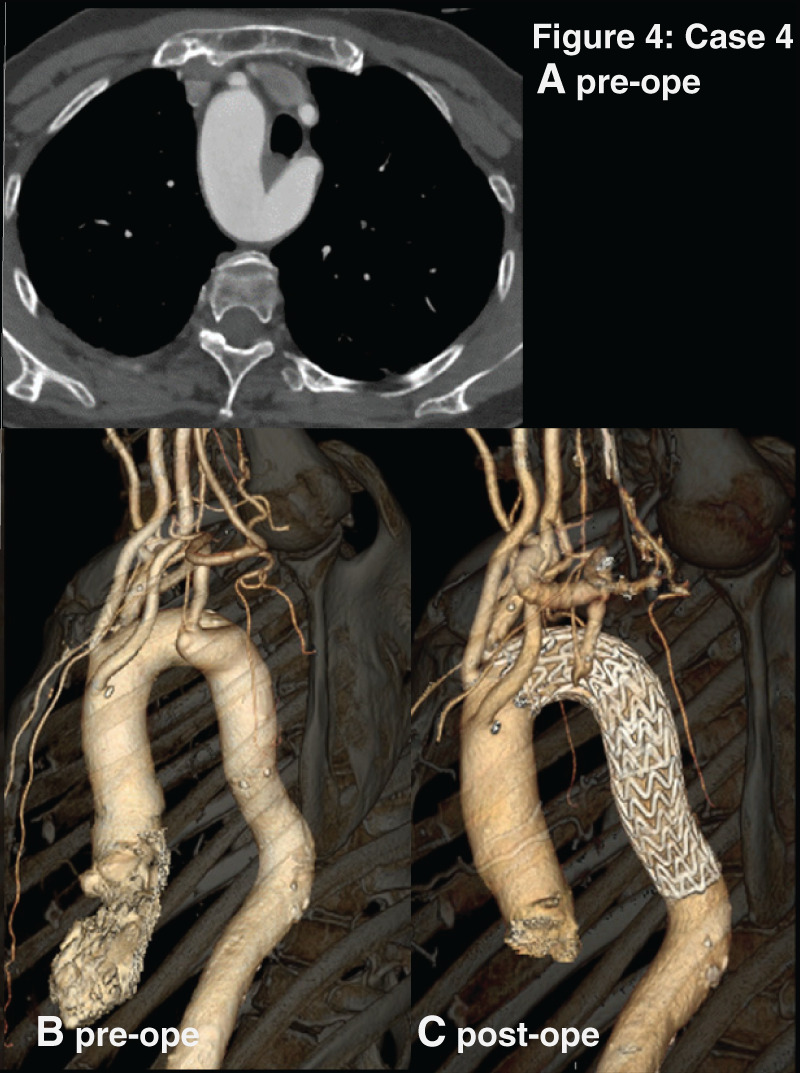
The figure 4 correspond to the images of Case 4 respectively. (A) Preoperative enhanced CT images, axial view. (B) Preoperative 3D-reconstructide CT. (C) Postoperative 3D-reconstructed CT. Case 4 are mirror-image RAAs with ALSCA. ALSCA, aberrant left subclavian artery; RAA, right-sided aortic arch
